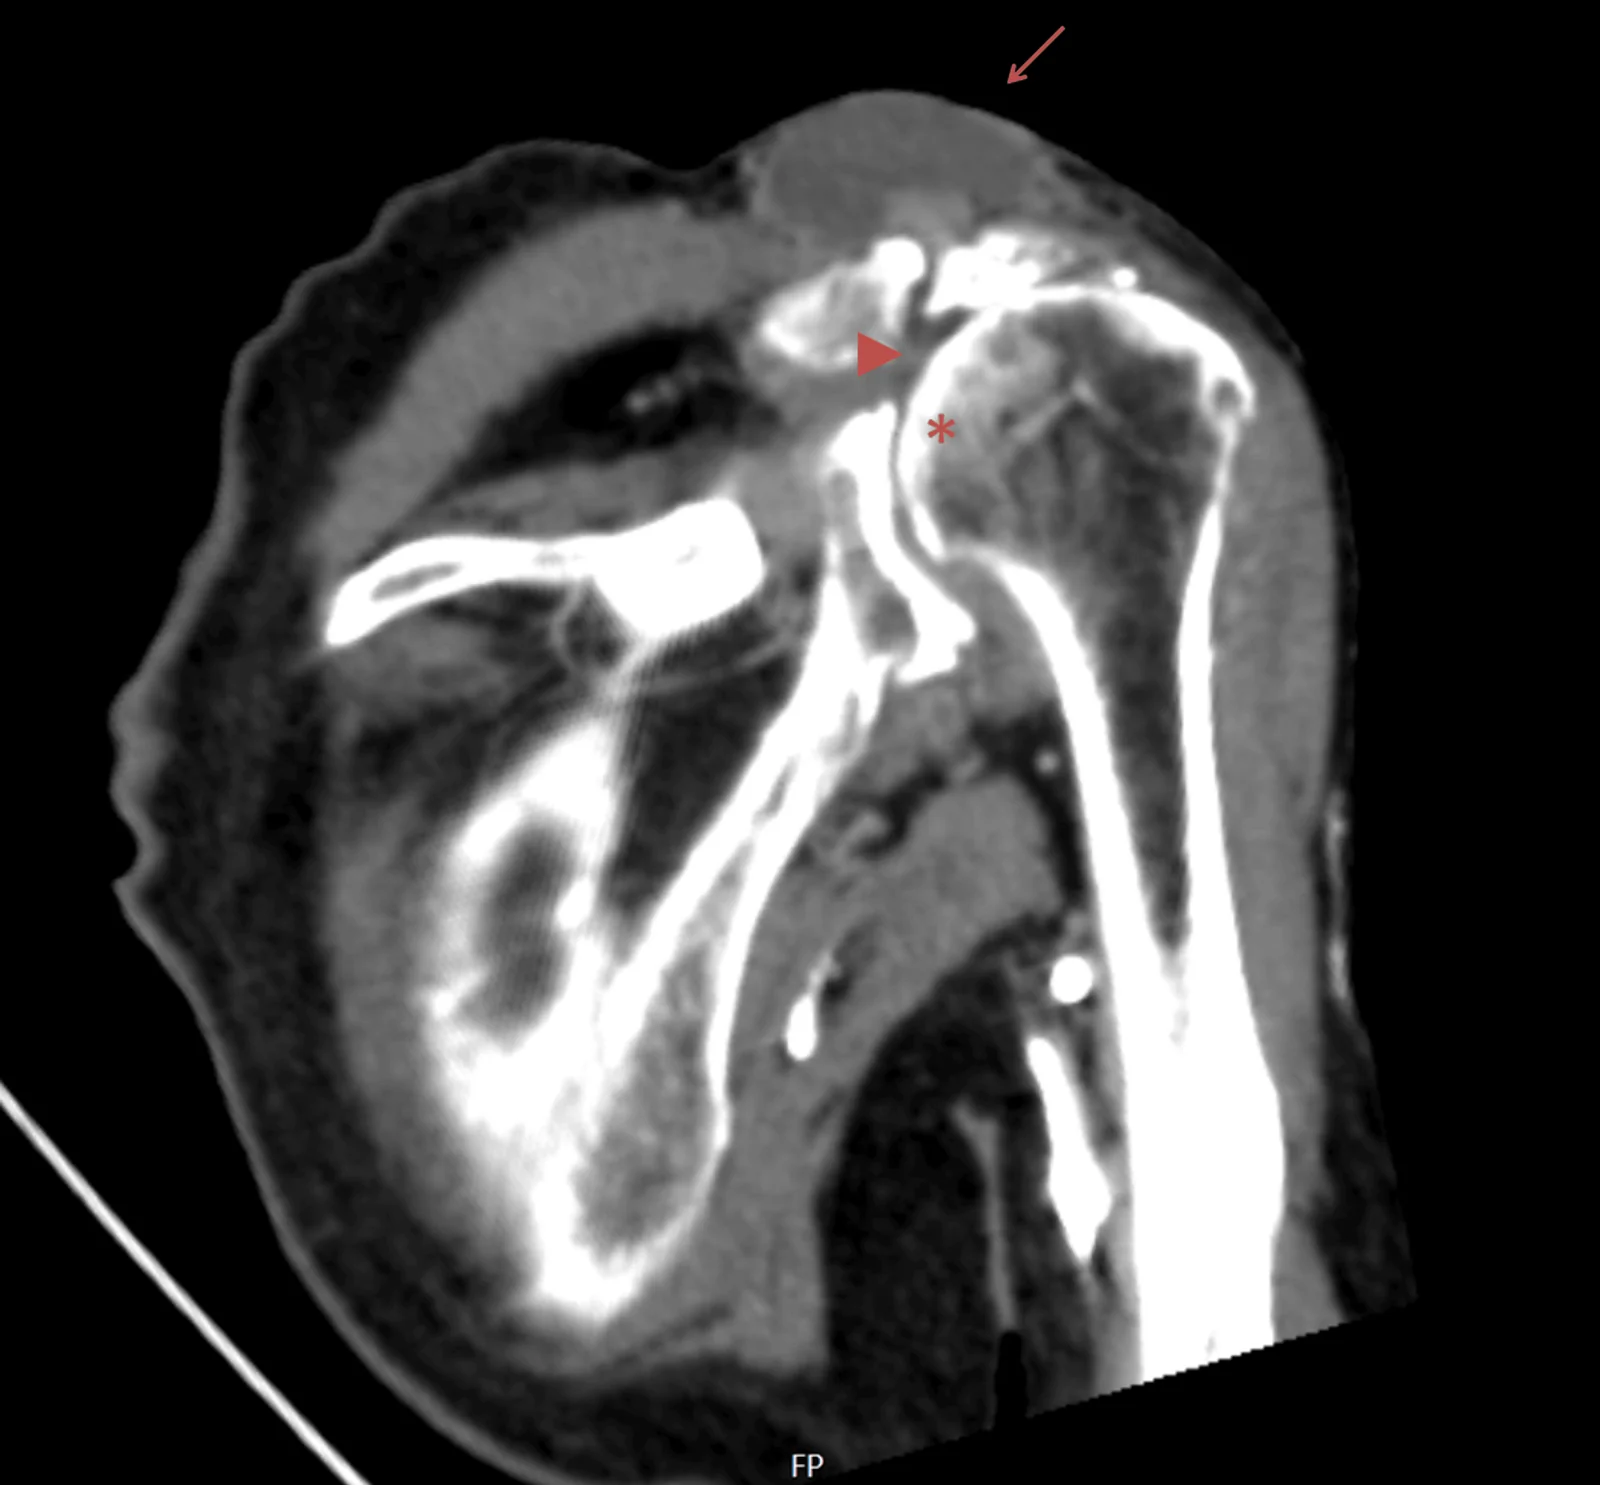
Coronal CT image of the left shoulder demonstrating an ACJ cyst (arrow), GHJ degenerative changes (asterisk), and a suspected superior fluid tract (arrowhead), compatible with the GS. ACJ, acromioclavicular joint; GS, Geyser Sign; GHJ, glenohumeral joint
